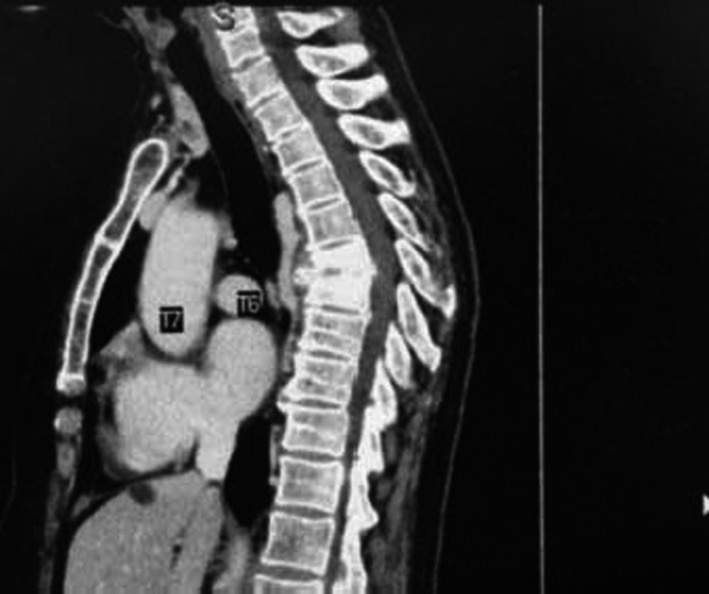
Thoracic CT scan, sagittal view, shows destruction of the body of T6, T7 vertebrae
